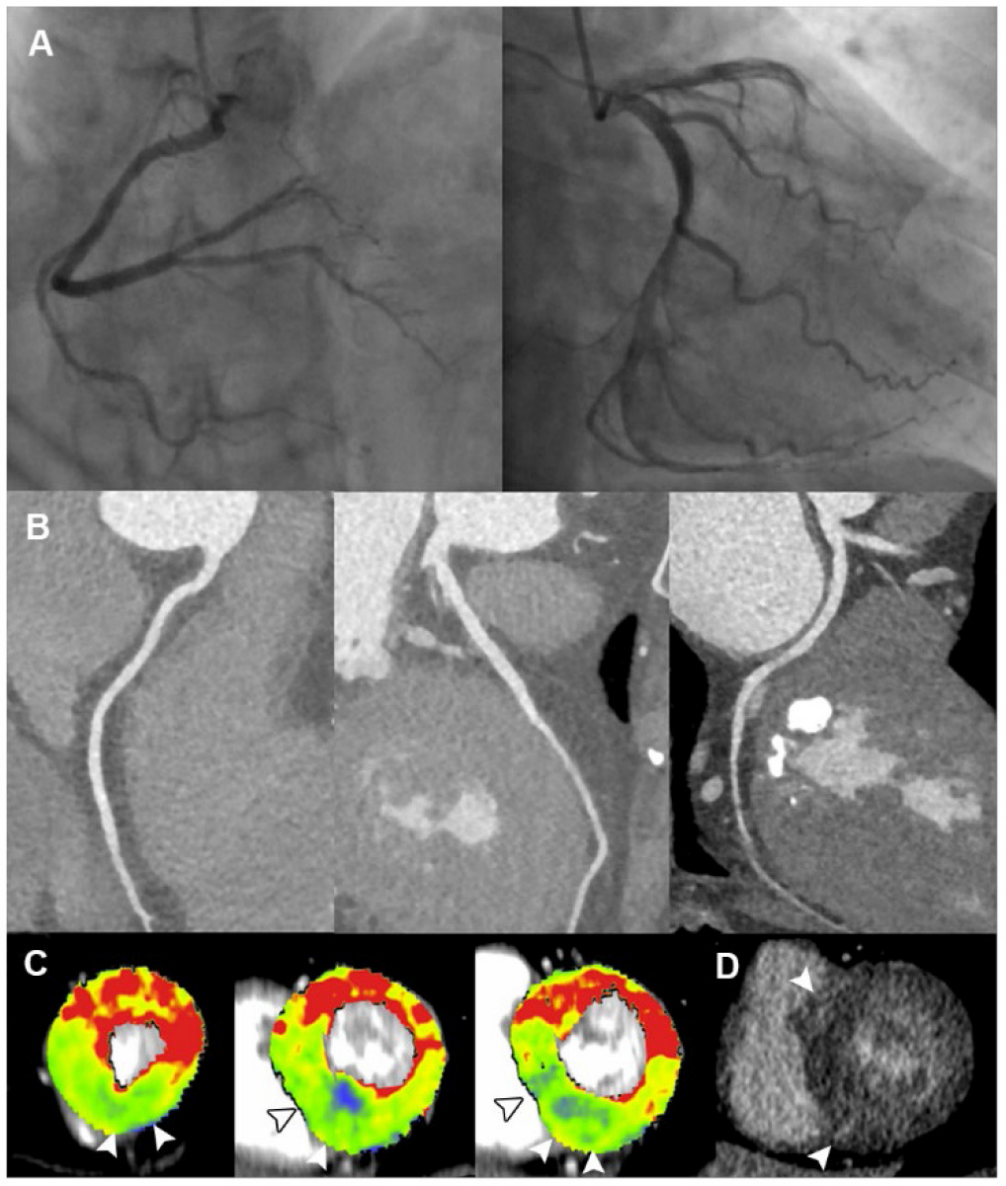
Representative case of cardiomyopathy in a 60-year-old female with ACS and non-obstructive ICA. (A,B) ICA and CCTA revealed normal coronary vasculature. (C) Short-axis views of CT-MPI showed significantly reduced myocardial blood flow at apical inferior, mid septal, mid inferior, basal septal as well as basal inferior segments, with focal myocardial hypertrophy (white arrowhead). (D) LIE image showed the focal intra-mural late enhancement at RV insertion points (white arrowhead). The above findings were consistent with the diagnosis of hypertrophic cardiomyopathy. ACS, acute coronary syndrome; CCTA, coronary computed tomography angiography; CT, computed tomography; ICA, invasive coronary angiography; LAD, left anterior descending; LAP, low-attenuation plaque; LIE, late iodine enhancement; MPI, myocardial perfusion imaging; MVO, microvascular obstruction; RV, right ventricle.
